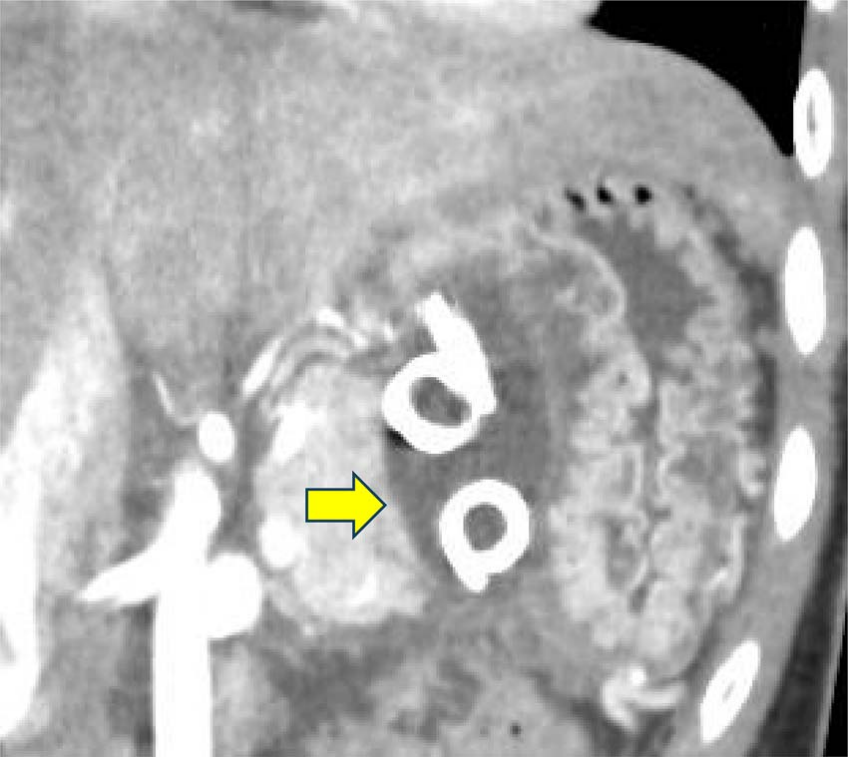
Contrast-enhanced CT on the 12th day. The pseudocyst in the omental bursa had shrunk. The arrow indicates the pseudocyst.
